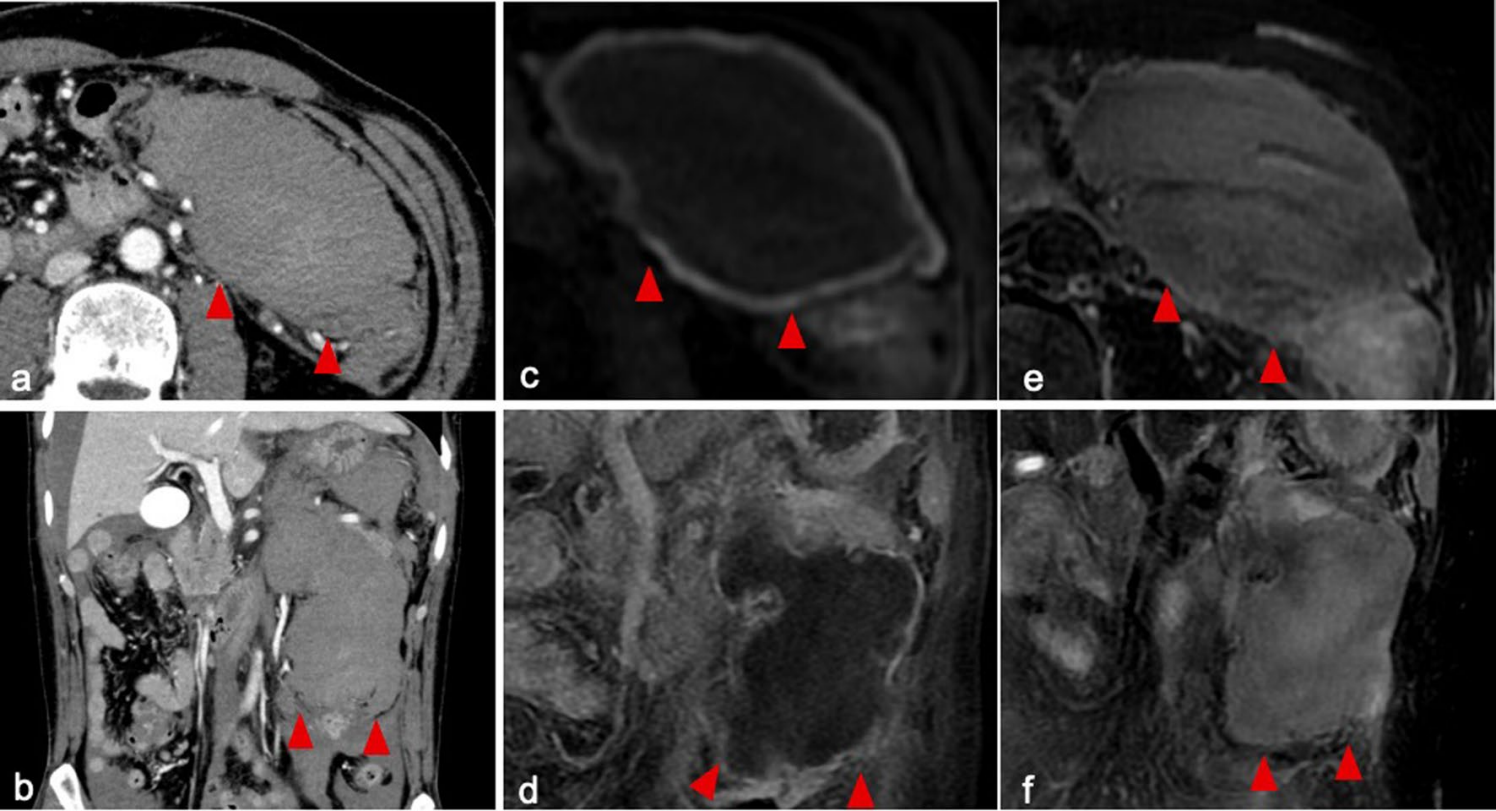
CT and MRI of the abdomen. a Contrast-enhanced CT revealed a large low-density area (arrow heads) in the left peritoneal space. b Coronal contrast-enhanced CT revealed a low-density area (arrow heads) in the left retroperitoneal space. Neither extravasation nor causative aneurysm was observed. c T1-weighted MRI revealed that the mass (arrow heads) was encapsulated by hyperintense rims. d Coronal T1-weighted MRI performed after gadolinium administration revealed no extravasation in the mass (arrowheads). e T2-weighted MRI showed homogeneously high intensity in the mass (arrowheads). f Coronal T2-weighted MRI showed homogeneously high intensity in the mass (arrowheads). *CT* computed tomography, *MRI* magnetic resonance imaging
